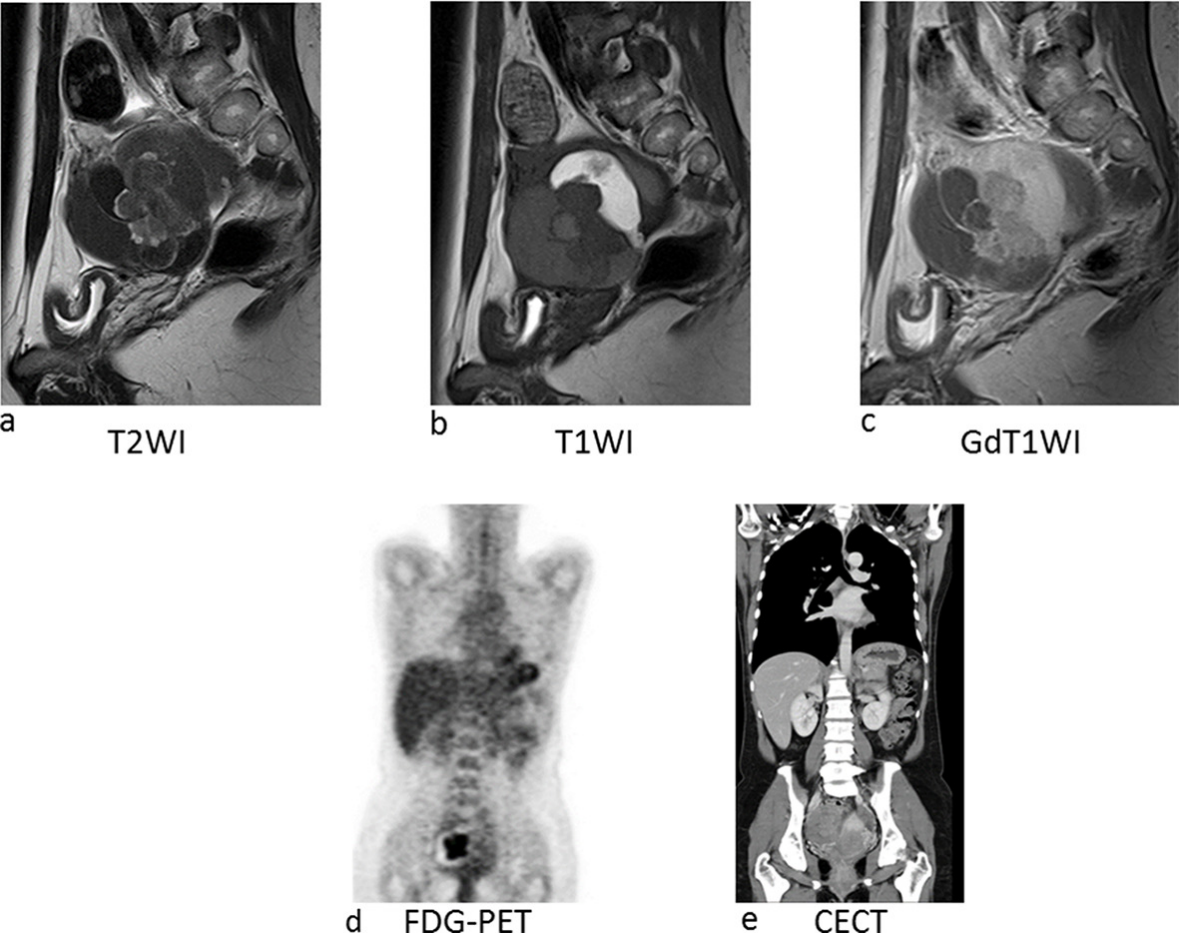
Imaging of Case 1. (a) Sagittal *T*_2_ weighted image shows multicystic tumour. Each locule shows different signal intensity and also include papillary lesion in the middle of the tumour. (b) On sagittal *T*_1_ weighted image, some of the locules showed high signal intensity, indicating haemorrhagic content within the cyst. (c) Gadolinium enhanced *T*_1_ weighted image, middle part of the locules and papillary lesion within the locule were well enhanced and indicated the presence of solid component. (d) FDG-PET image shows strong uptake with irregular shape at the right side of the pelvis. According to the contrast enhanced CT image (e), those uptake corresponds to the right ovarian tumour, especially, solid component within the tumour. FDG-PET, fluorodeoxyglucose positron emission tomography.
